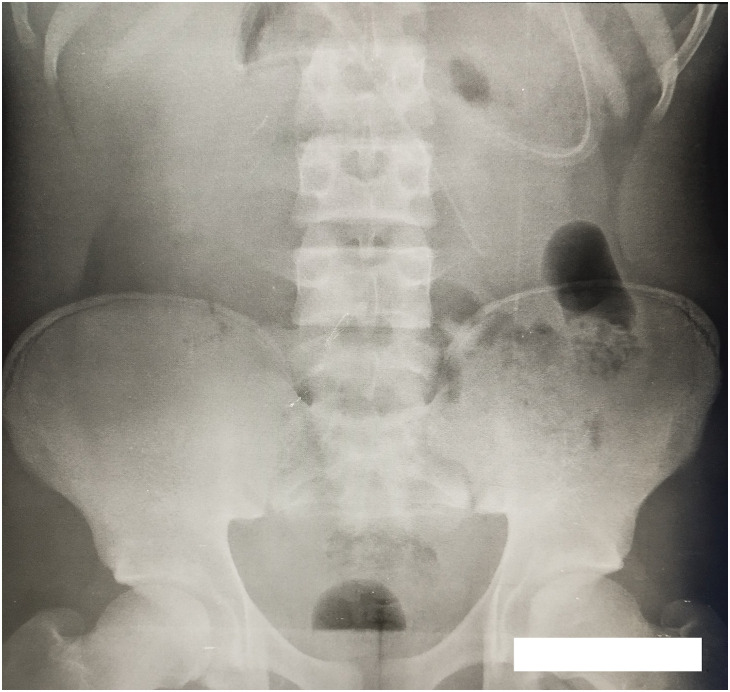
Plain abdominal X-ray showing hydroaeric levels.
